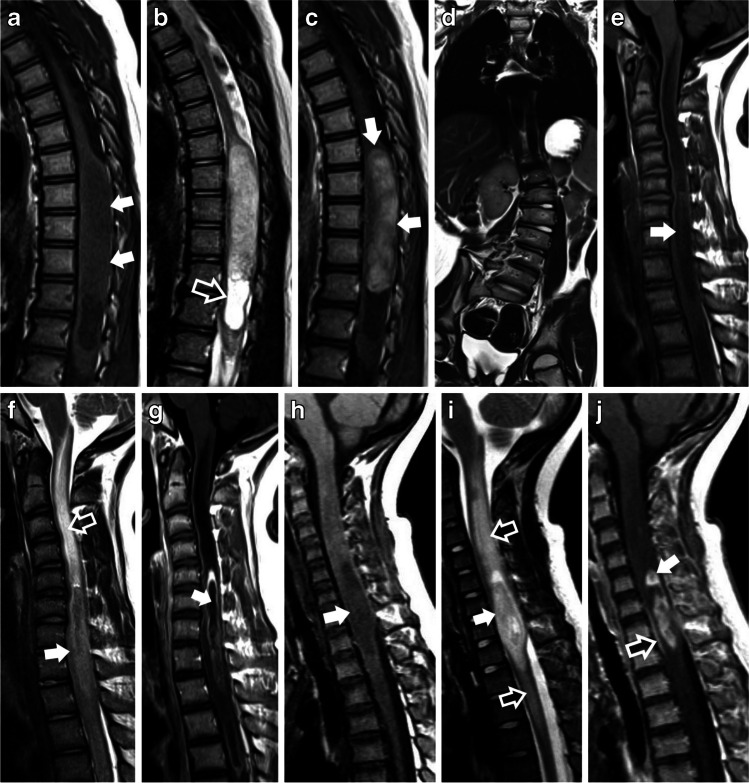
(a – j) Examples of High-grade vs Low-grade astrocytomas. (a – d) Pilocytic astrocytoma in a 4-year-old boy who presented with a 2-month history of progressive right lower leg weakness and gait difficulty. a) Sagittal T1 and b) Sagittal T2 show an expansile, solid, intramedullary mass in the thoracic cord, extending from T7 to T12 (solid white arrow in a), with an associated caudal syrinx (white hollow arrow in b) and spinal canal widening. c) Sagittal T1 after contrast administration demonstrates a sausage-shaped tumor with nodular/mass-like enhancement and well-defined/crisp borders (Type 1A) (solid white arrow). d) Coronal T1 composition of the entire spine shows marked levo-convex scoliosis of the thoracolumbar spine from T10-L5. (e – g) Diffuse midline glioma H3 K27-altered in a 13-year-old girl who presented with 3 weeks of progressive weakness in her arms and legs. e) Sagittal T1 and f) Sagittal T2 TSE images show an expansile, ill-defined, intramedullary mass, extending from C5 to T2 (solid white arrow). The mass is associated with edema which extends rostrally to the lower medulla (white hollow arrows in f). g) T1 FLAIR after contrast administration shows a nodular/mass-like enhancing pattern with ill-defined/shaggy borders (type1b) and central non-enhancement (solid white arrow). (h – j) Diffuse pediatric-type High-grade glioma in a 5-year-old male with a rapid onset of right-hand weakness with right-sided upper back and shoulder pain. h) Sagittal T1 and i) Sagittal T2 TSE show an expansile, well-defined, solid-cystic/necrotic, intramedullary mass in the cervico-thoracic cord, extending from C5 to the upper endplate of T2 (solid white arrow in h and i). There is associated edema extending rostrally to the lower medulla and caudally to the level of T5 (white hollow arrows). j) Sagittal T1 TSE, obtained after contrast administration, shows a nodular/mass-like enhancing pattern with a lobulated appearance, and well-defined/crisp borders (type 1a) (solid white arrow). Note the associated leptomeningeal enhancement (hollow white arrow)
